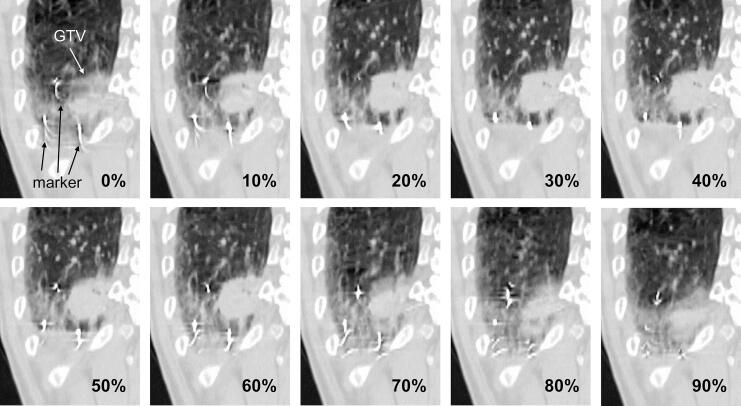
4D-CT images of the case with the lowest ${GTV}_{union}^{shift}$ V_100%_. Spherical fiducial markers with a diameter of 2 mm appear approximately 10 mm in length or are divided into several parts owing to severe artifacts. *Abbreviations*: 4D-CT, four-dimensional computed tomography; GTV, gross tumor volume.
